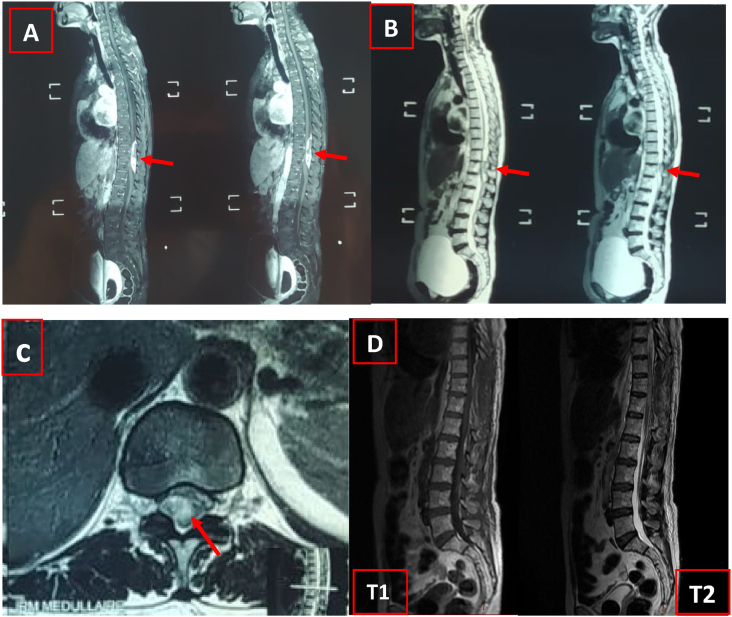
(A) T1 weighted Magnetic resonance imaging in sagittal view demonstrating spontaneous spinal epidural hematoma (SSEH) from T10 to T12 indicated by the arrow. (B) T2 weighted MRI images in sagittal view indicating SSEH (Arrows) (C) T2 weighted MRI images in transversal view showing the SSEH (Arrow) (D) T1 et T2 weighted MRI images performed after surgery showing the complete evacuation of hematoma.
